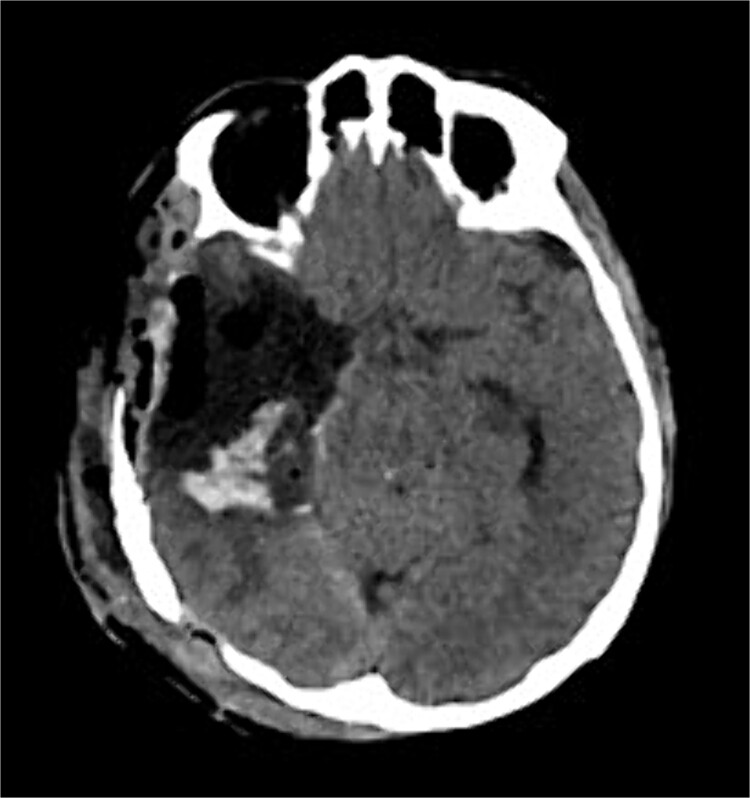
Postoperative axial CT shows postoperative changes with maximum safe resection of the lesion. CT = computed tomography.
